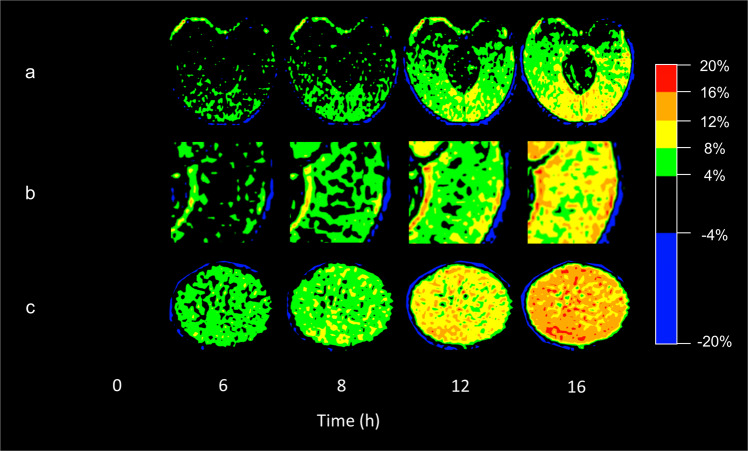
Time course of D_2_O uptake into sweet cherry fruit as viewed by magnetic resonance imaging. Virtual sections through whole fruit (a), detailed view (b, c) of equatorial cross section through the shoulder (b) and of tangential section through the stylar scar region (c). For sketch and nomenclature of the different regions see Fig. 1, (a, b, c) correspond to (a-S3), (c-S3), (c-S5) in Fig. 1, respectively. The D_2_O label increases as colour changes from black to green to yellow and red
